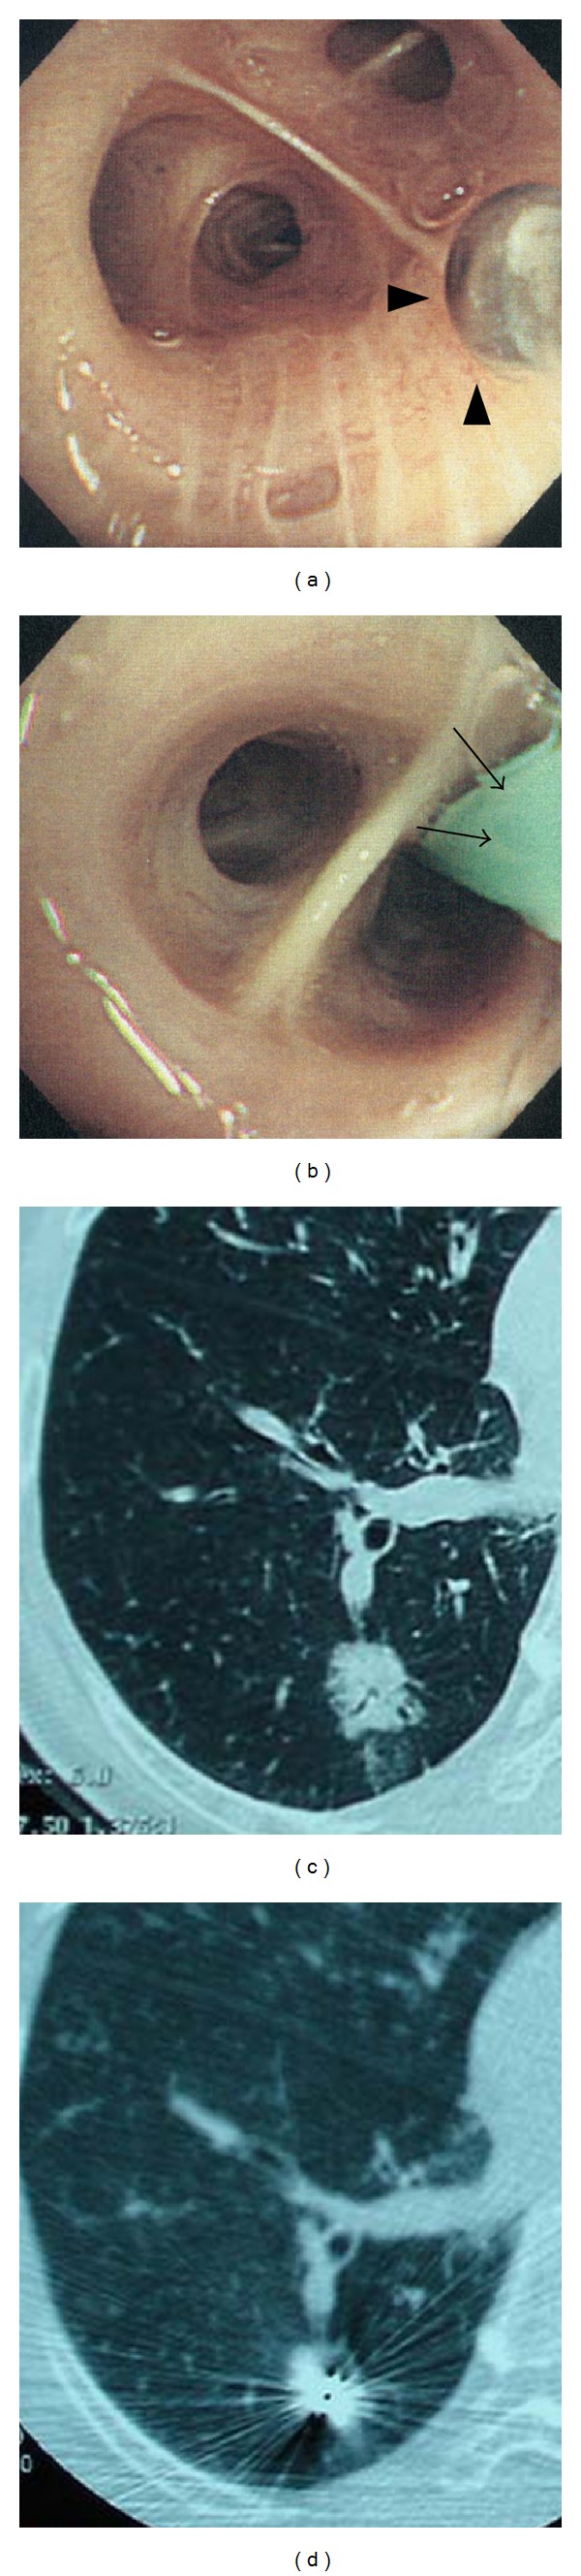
((a), (b)) Flexible fiberoptic bronchoscope (Olympus; Tokyo, Japan) BF 260 (outer diameter: 4.9 mm, forceps channel: 2.0 mm). 5 Fr cooled catheter tip was inserted under fluoroscopic guidance. (a) The tip of the electrode (arrowheads). (b) Shaft of the electrode (arrows). ((c), (d)) Low-dose CT guidance was performed to correlate the location of the catheter tip in the lung tumor. (c) Peripheral lung tumor (ground-glass opacity) (arrow) on low-dose CT image. (d) Halation around tumor on CT image reflected the catheter tip.
